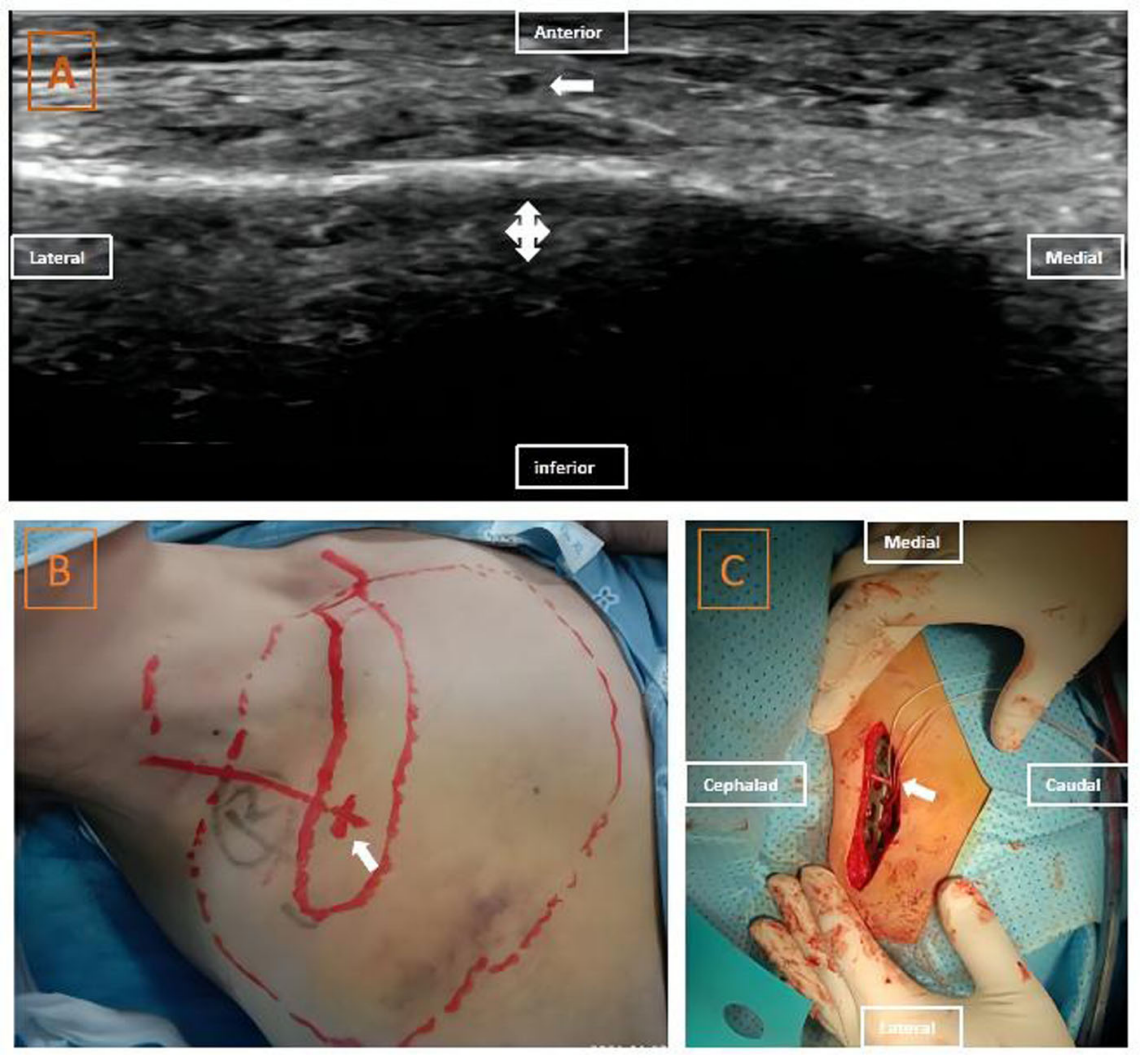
Ultrasound images, projection on the body surface, sensory block area and intraoperative view of supraclavicular nerve in case 2: (A) ultrasound images of the supraclavicular nerve anterior to the clavicle; (B) projection of the supraclavicular nerve on the body surface and the sensory block area. (C) intraoperative view of the supraclavicular nerve. The thick white arrow indicates the supraclavicular nerve; the cross arrow indicates the clavicle.
